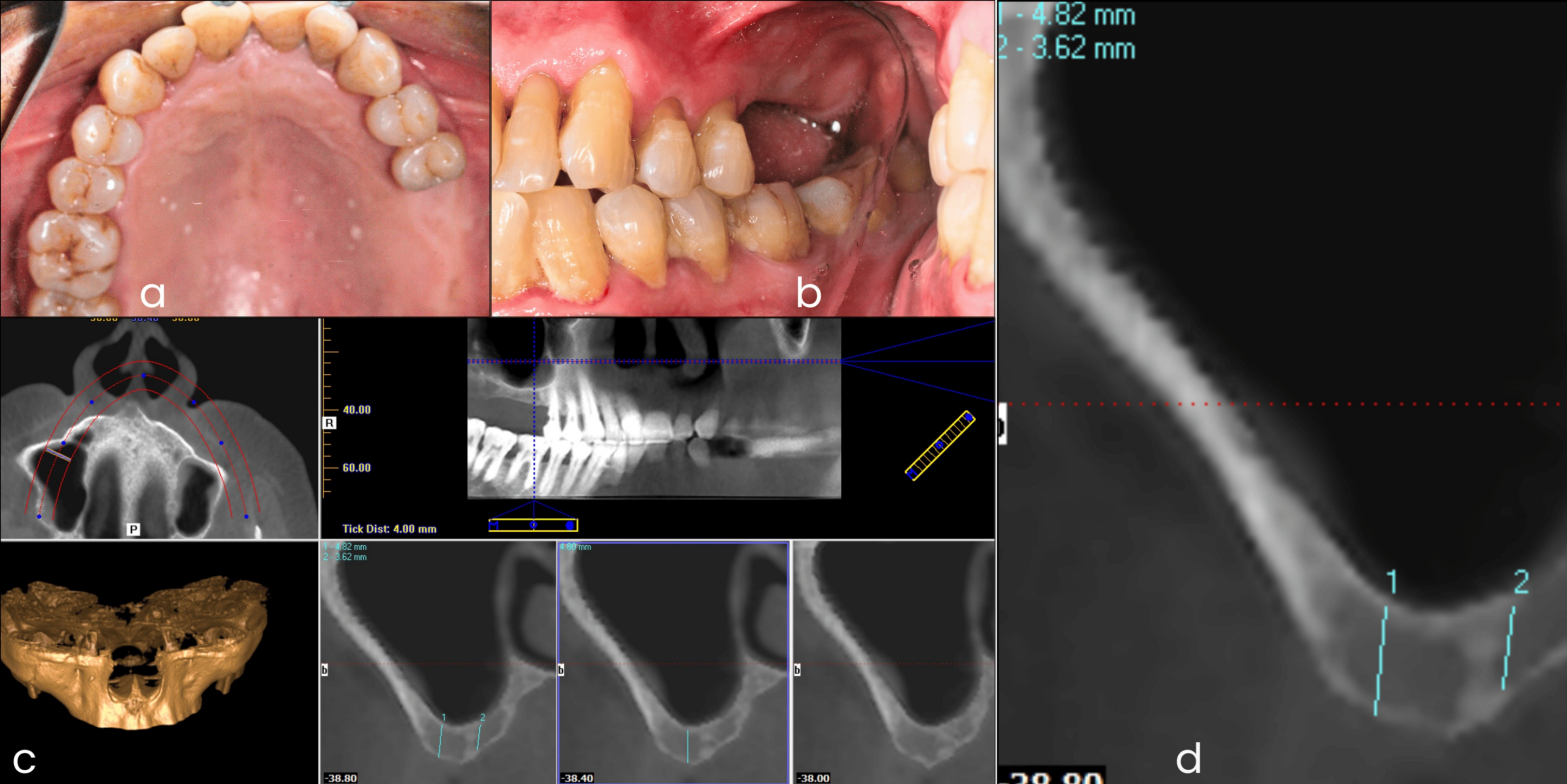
Photographs of the diagnostic phase including cone-beam computed tomography (CBCT) evaluation. (a) Intraoral maxillary arch occlusal mirror view. (b) Intraoral lateral mirror view. (c) Diagnostic CBCT image. (d) CBCT image assessment showing deficient bone height.
